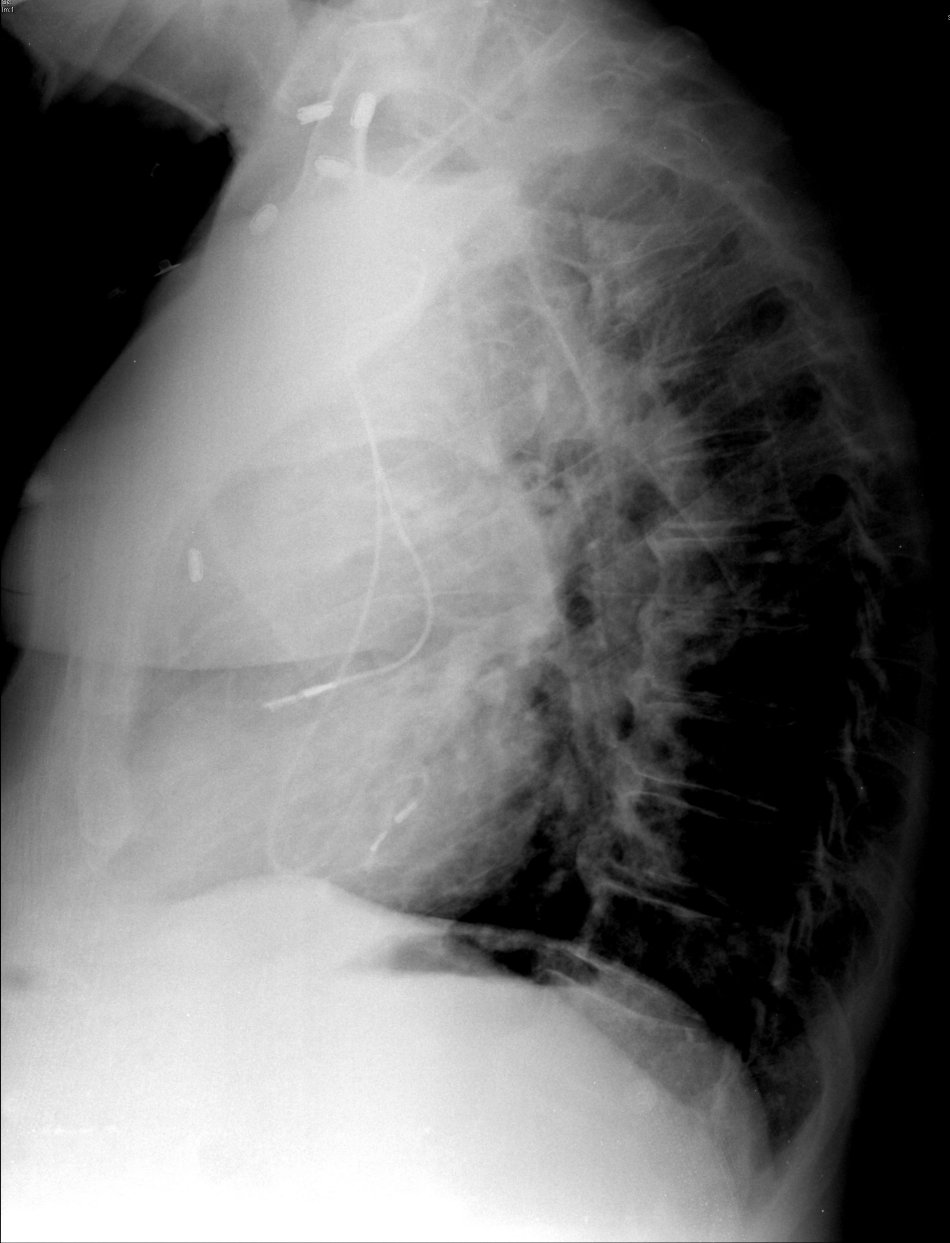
Chest radiograph lateral projection showing the ventricular lead to be pointing posteriorly, suggesting a left ventricular site.
